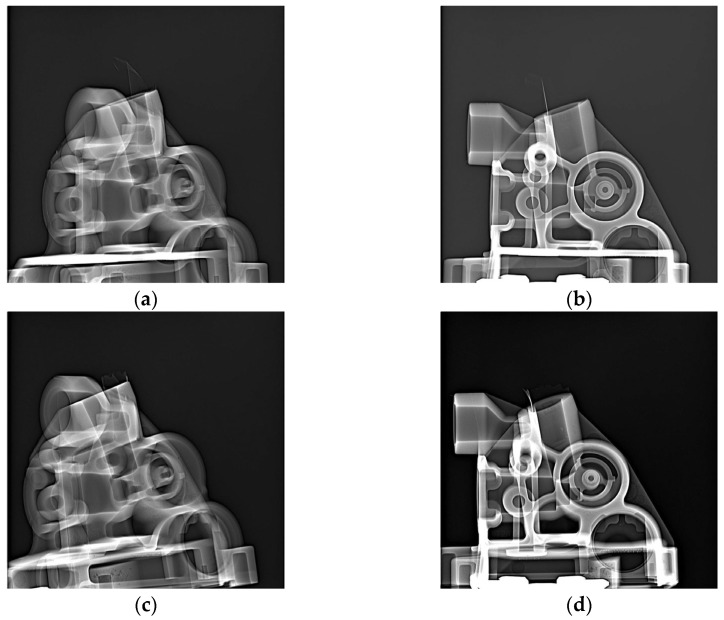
X-ray inspection for nominal (about 26 mm) value of biscuit thickness in specific sockets: socket C1: (a) area A—rate value 1, (b) area B—rate value 2; socket C2: (c) area A—rate value 2, (d) area B—rate value 2.
